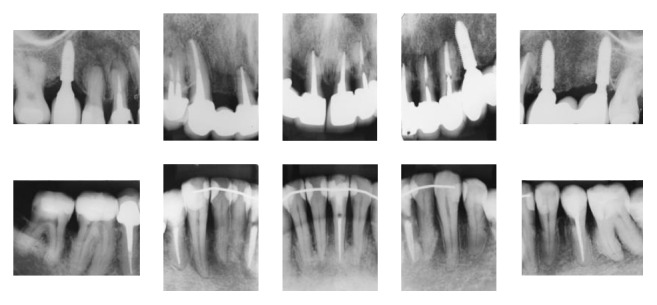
Posttreatment periapical radiographs 1 year and 11 months after debonding.
